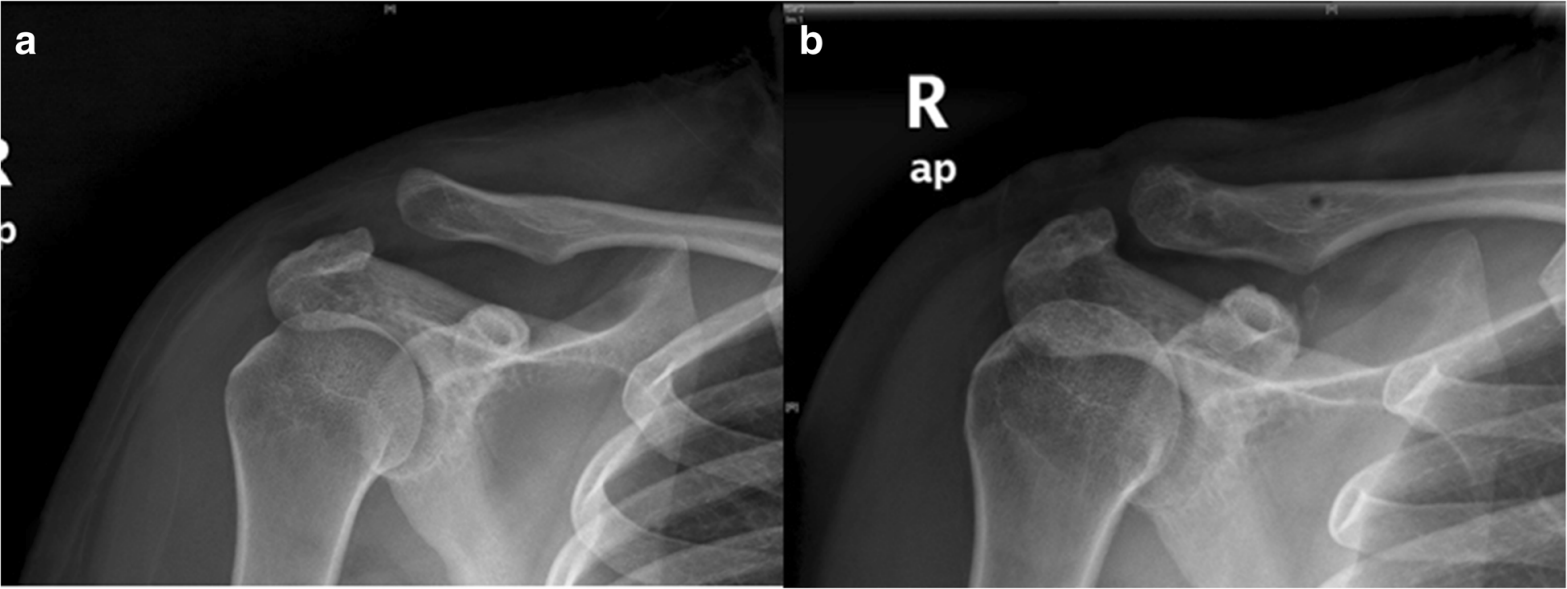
a X-rays of AC joint dislocation before surgery. b X-rays of AC joint dislocation after surgery reconstructing the coracoclavicular and acromioclavicular ligaments with the allogenic tendon
